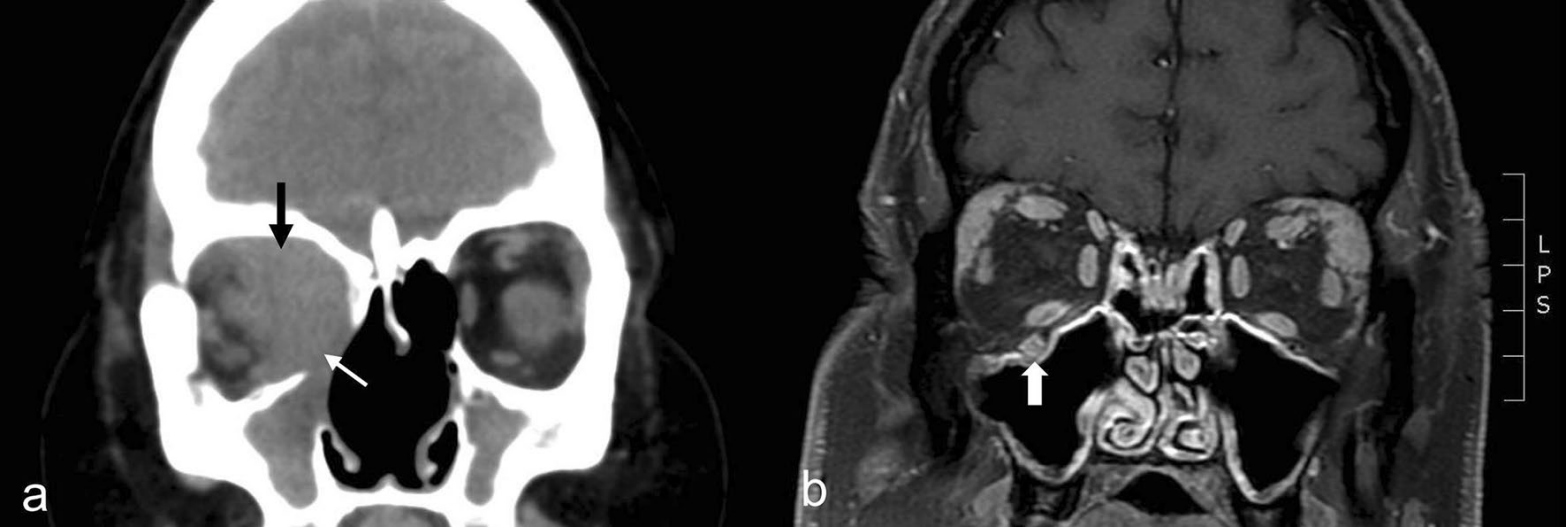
Representative images of granulomatosis with polyangiitis (GPA) and IgG4-related ophthalmic disease (IgG4-ROD). (A) Coronal unenhanced computed tomography scan shows diffuse, homogeneous, retrobulbar mass affecting extraconal and intraconal space of the right orbit (black arrow). Note nasal septal destruction and the contiguous sinus involvement with orbital wall and orbital floor destruction, characteristic of GPA (white arrow). The radiologic diagnosis was GPA, compatible with the clinical diagnosis. (B) Asymmetric, bilateral lacrimal gland enlargement is seen on coronal, fat suppressed T1-weighted contrast-enhanced magnetic resonance image. Right infraorbital nerve enlargement (white arrow) is reported as a more specific finding of IgG4-ROD, and the radiologic diagnosis was IgG4-ROD.
